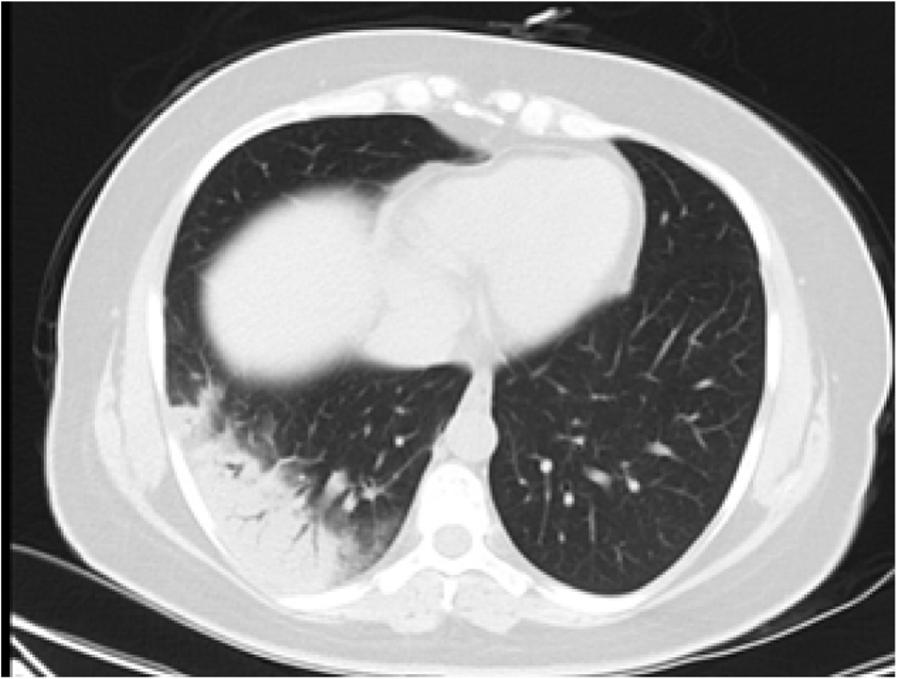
COVID-19 pneumonia: thin section CT shows non segmental parenchymal consolidation with airbronchogram in right lower lobe.
